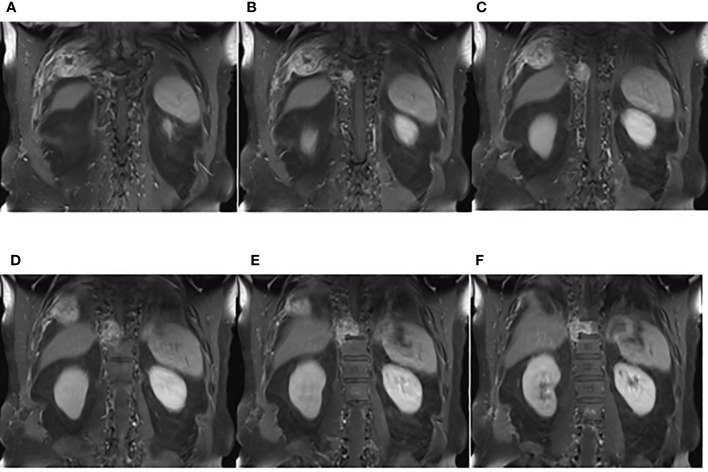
The coronal plane of MRI findings on December 6, 2019. (A–F) Metastasis of the thoracic vertebrae and chest wall on the coronal planes of enhanced T1-weighted MRI.
